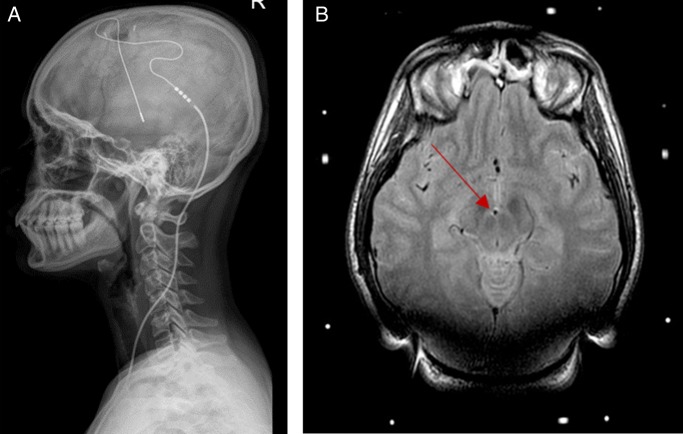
Deep brain stimulator for chronic cluster headache. (A) Skull X-ray shows placement of occipital nerve stimulation electrodes and leads. (B) Postoperative MRI brain showing occipital nerve stimulation lead in right ventral tegmental area (arrow).
